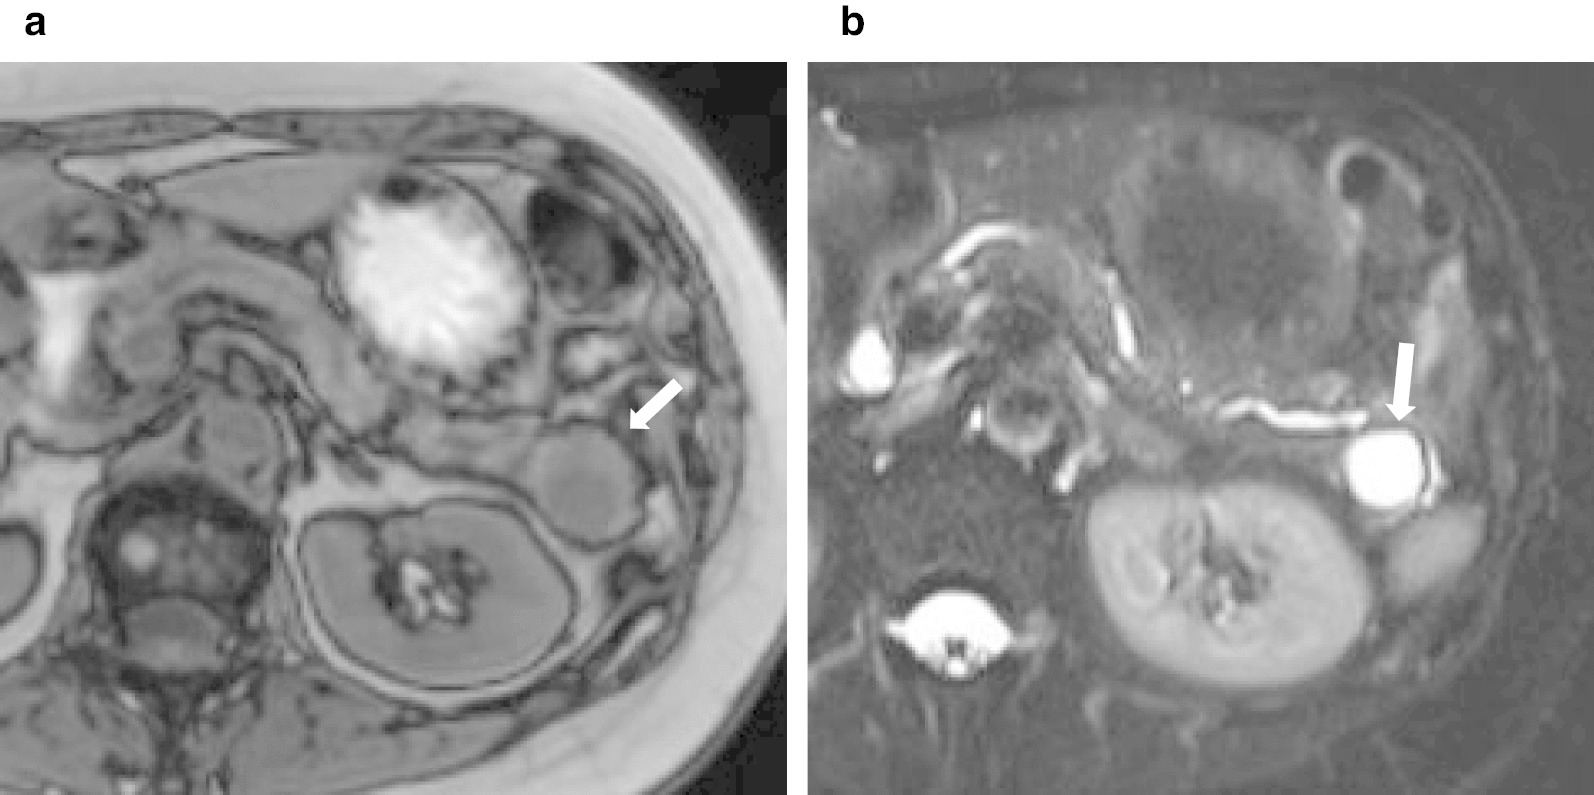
Magnetic resonance images (MRI) revealed that the cystic component showed a low signal intensity (arrow) on T1-weighted images (a) and a high signal intensity (arrow) on T2-weighted images (b)
